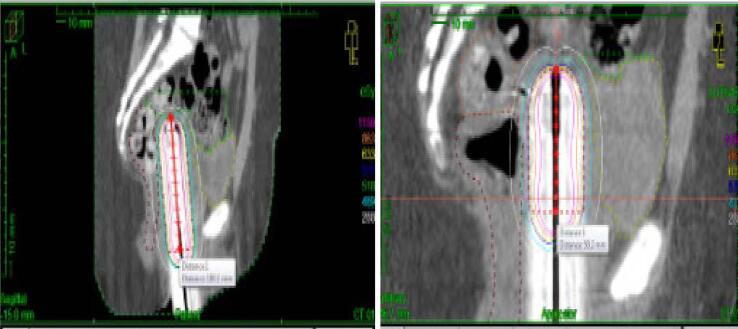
Vaginal Brachytherapy Treatment Plan The image on the left is a sagittal CT image with the initial plan treating the whole vagina followed by a boost field on the right to a smaller area covering the post-operative bed.
